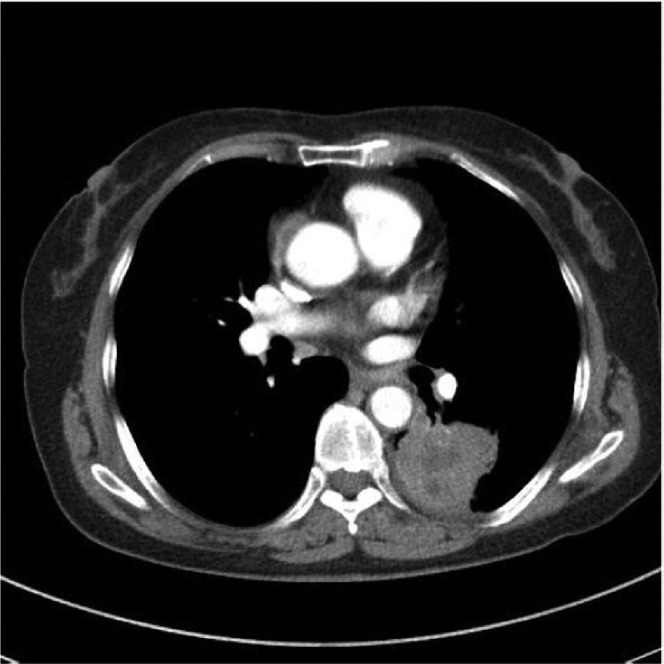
Computed tomography demonstrates a lesion at left lower lung.
